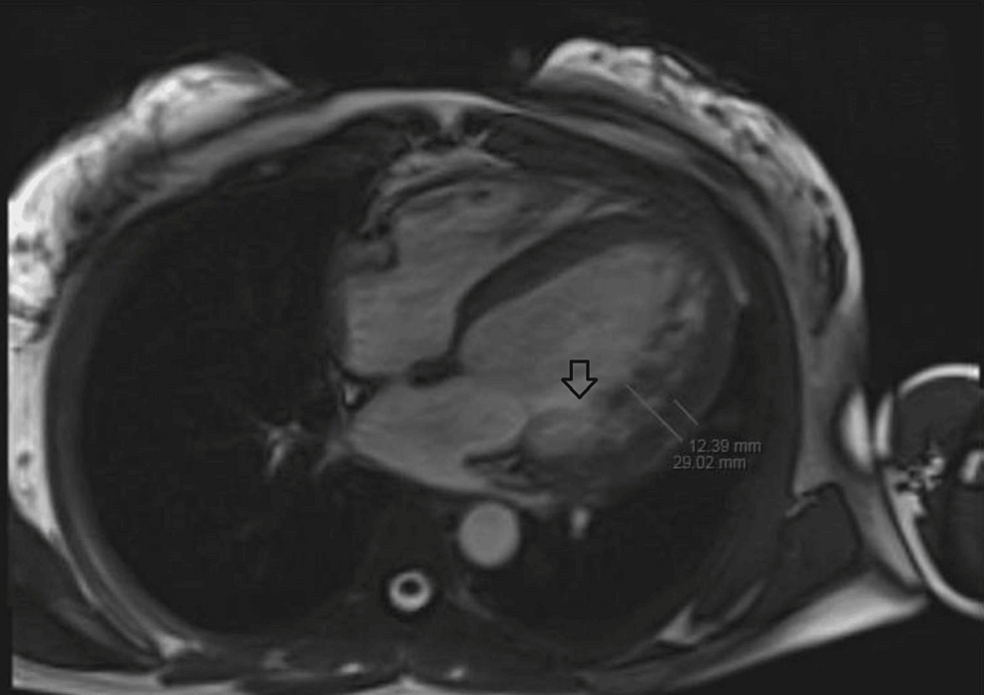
Arrow pointing towards increased left ventricular thickness and trabeculations on cardiac MRI. MRI: magnetic resonance imaging
